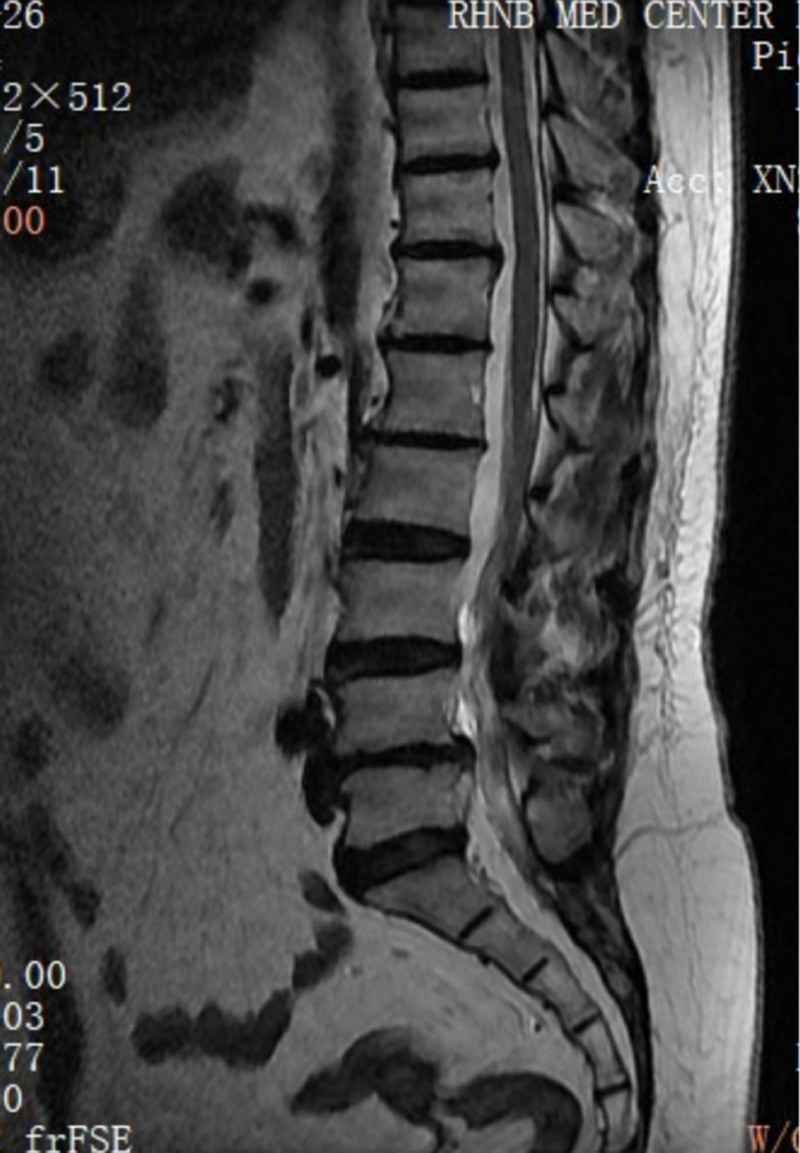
MRI sagittal view showing lumbar spondylolisthesis at the L4/5 level. MRI = magnetic resonance imaging.
